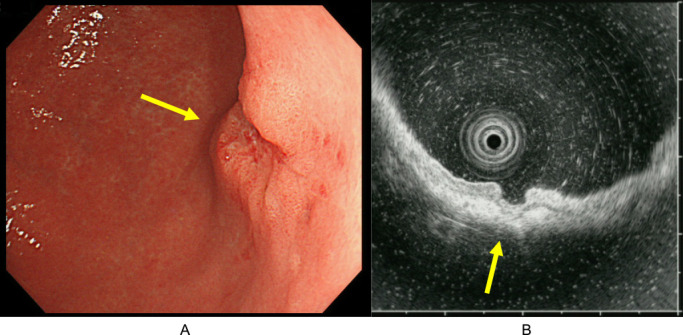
Gastric endoscopy and endoscopic ultrasonography findings. (A) Endoscopy revealed superficial adenocarcinoma (arrow) on the posterior wall in the corpus of the stomach. (B) In the endoscopic ultrasonography, the tumor was suspected to have invaded the second layer (mucosa) with partial invasion into the third layer (submucosa) (arrow).
